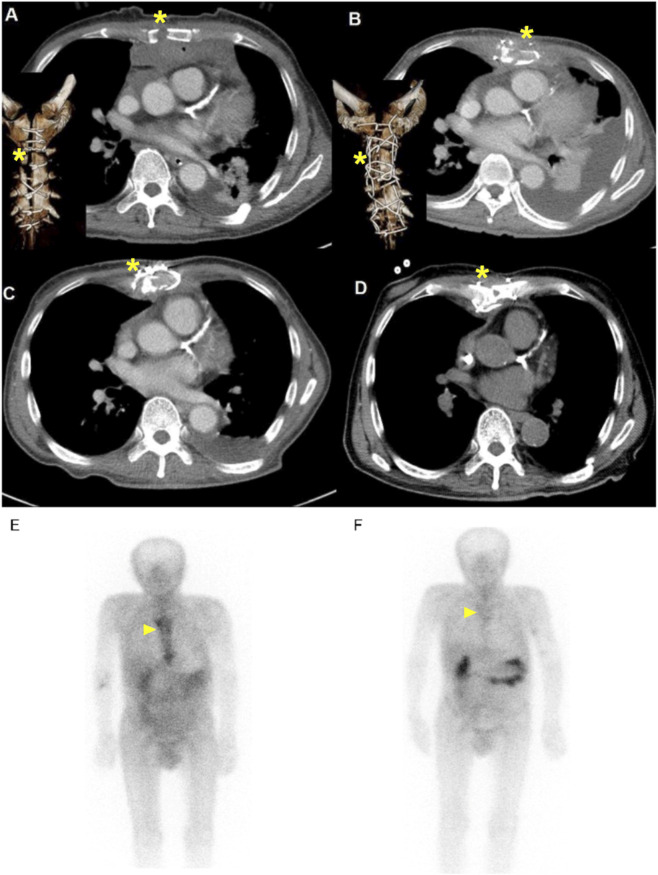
Serial images of chest computed tomography and Ga^67^ inflammation scans of case 1 using 0.5 g Ezechbone® Granule CBS-400. (A) 14th post-operative day after 1st operation (emergency CABG). Dehiscent sternum with wire cut-through cleft at middle sternal body (yellow asterisk). (B) 2 months after the 2nd operation with Robicsek fixation method. (C) 4 months after 2nd operation with new bone ingrowth in wire cut-through cleft. (D) 10 months after 2nd operation with complete bone union of sternotomy and wire cut-through cleft. (E) Sternal osteomyelitis indicated by increased radioactivity at the midline anterior chest area (yellow arrowhead) before 2nd operation. (F) Complete resolution of sternal osteomyelitis indicated by disappearance of radioactivity at the midline anterior chest area 3 months after 2nd operation.
